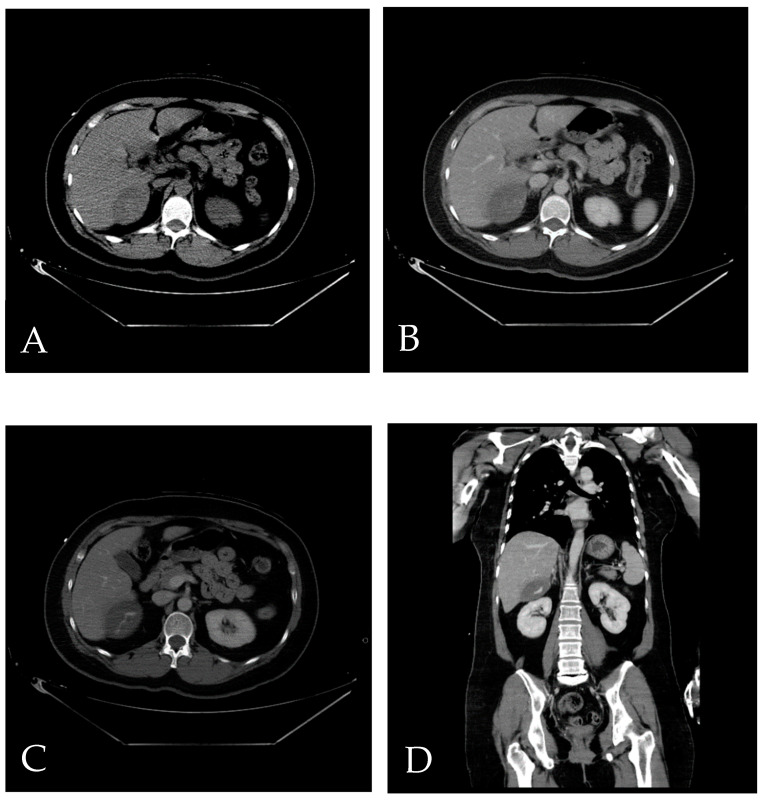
A 44-year-old woman indicated to further TAE was involved in a traffic accident [1]. (A) Axial-view CT without contrast reveals a well-defined lesion in the region of segments 6 (S6) and 7 (S7) of the liver, with a Hounsfield Unit (HU) value approximately around 60, indicative of a hematoma. (B,C) Axial view CT with contrast demonstrates contrast extravasation, highlighting the presence of vascular leakage. (D) The reconstructed coronal view CT with contrast provides a clearer visualization of the lesion, elucidating its precise location.
